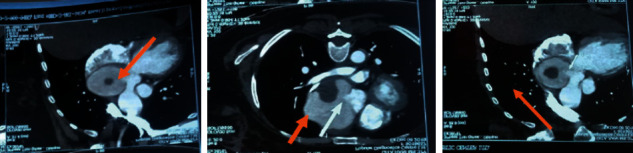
CT angiogram of the heart and great vessels. Large eccentric structure is noted in the root of the ascending aorta having fistulous communication. A hypodense area is noted within the structure. The structure is compressing the corresponding superior vena cava and left ventricular outlet or aortic root (7.5 mm), suggestive of large aneurysm at the aortic root containing the thrombus. Photo: courtesy of the patient.
